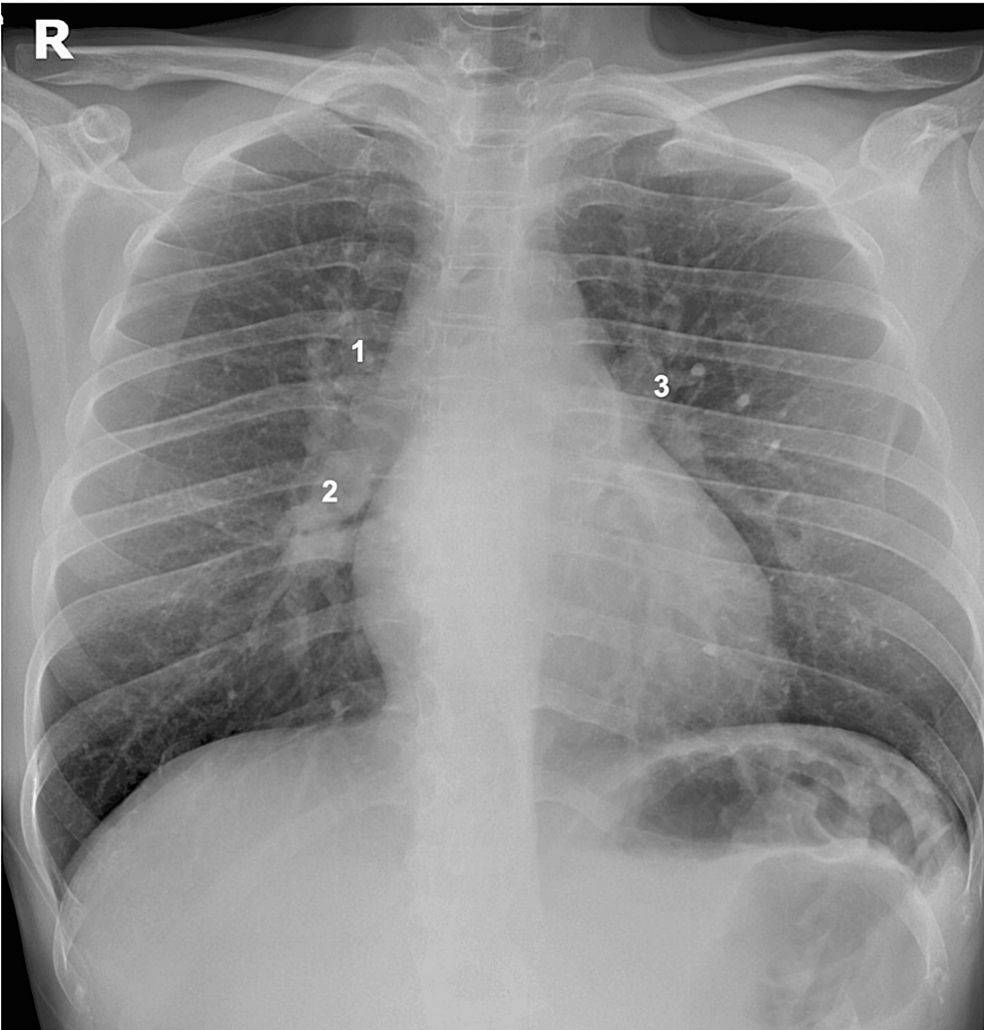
A PA chest x-ray revealing the classic pattern of sarcoidosis: 123 sign or Garland's triad. The characteristics of this sign include mediastinal enlargement, usually right paratracheal (1) and bilateral hilar nodes, on the right (2) and left (3), due to lymphadenopathy. PA, posteroanterior
